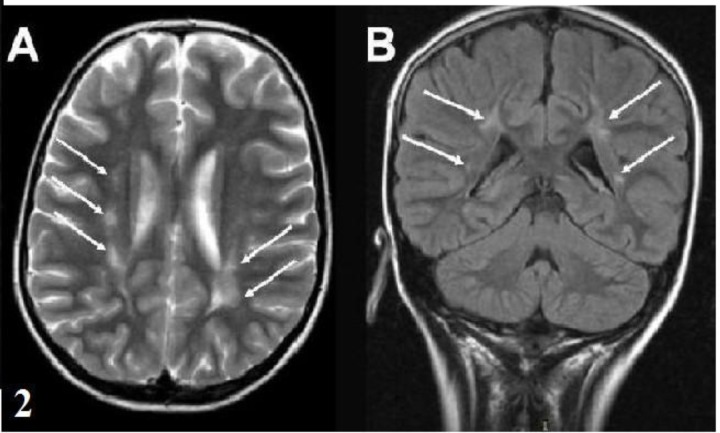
(A,B) Both T2-weighted axial image and the FLAIR coronal image show confluent hyperintense lesions in the bilateral periventricular-subcortical white matter. These lesions persist in the follow-up images (Patient 2).
